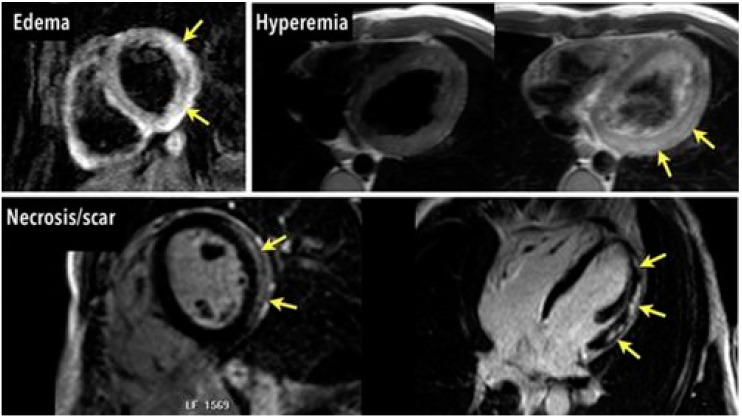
Myocarditis. Myocardial “Edema” identified using T2-weighted imaging. “Hyperemia” identified with early gadolinium enhancement. “Necrosis/scar” identified with late gadolinium enhancement. Yellow arrows are shown to indicate the respective re-gions of abnormal myocardial enhancement in myocarditis [45]
